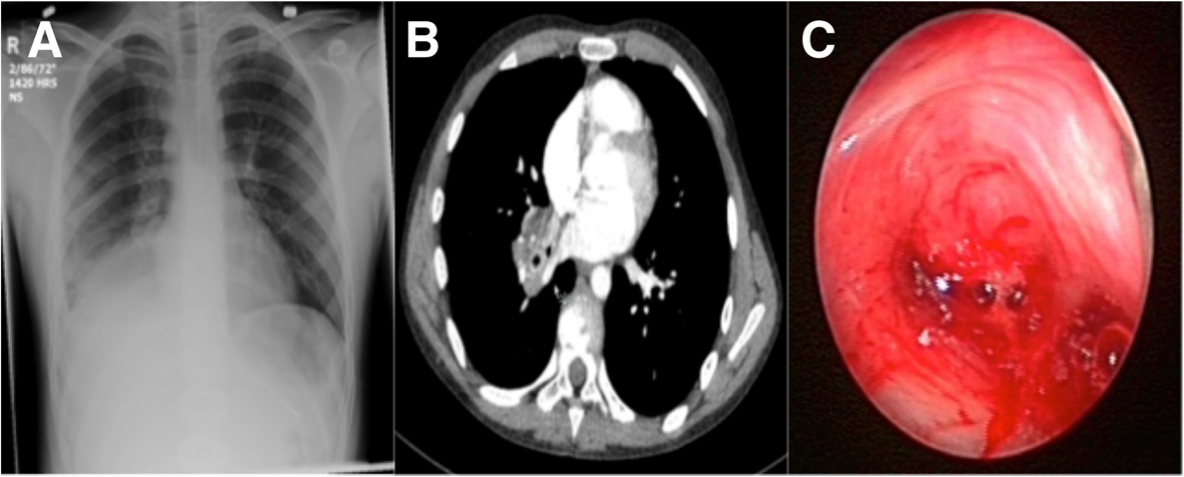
Case 1 - Posteroanterior (PA) chest x-ray showing complete right lower lobe opacification (a). CT of the chest showing right perihilar parenchymal opacification with partial collapse of the right lobe bronchus (b). Endoscopic view of extensive granulation tissue in the right main stem bronchus and excessive bleeding (c)
